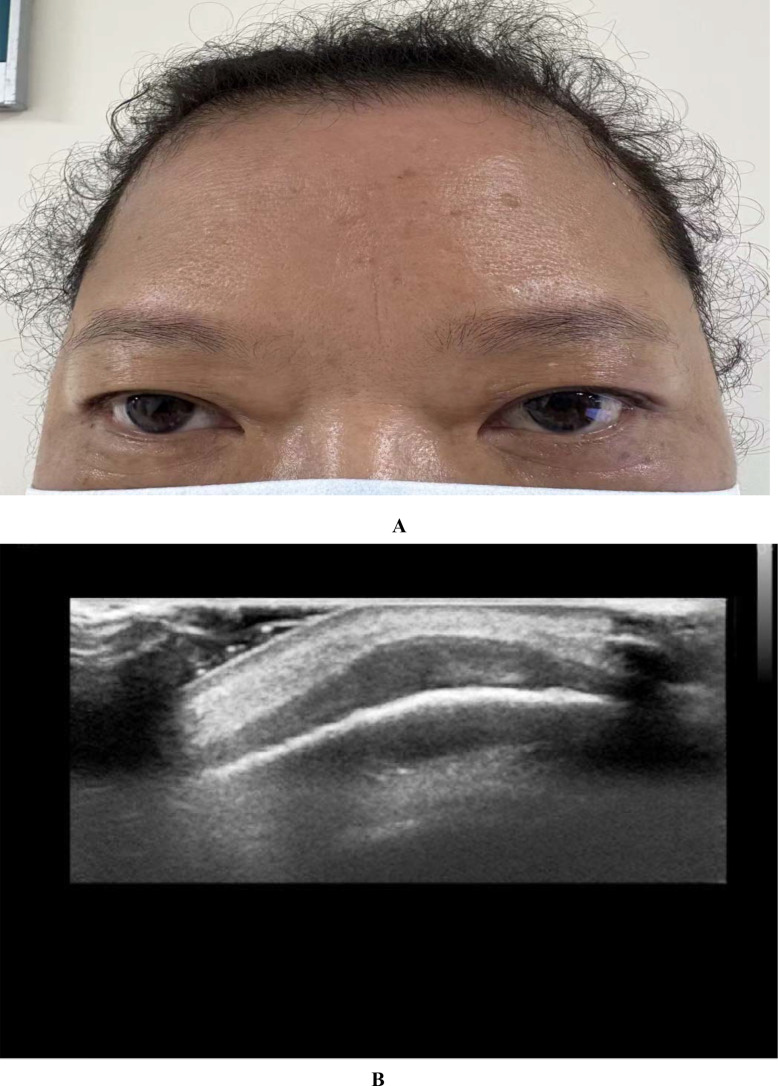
The palpable mass was almost completely resolved, and the edema around the right eyelid disappeared, with significant improvement in symptoms (A). After 4 cycles of Fulvestrant combined with Darsalizumab treatment, a follow-up superficial tissue ultrasound showed thickening of the subcutaneous layer in the left frontal area, with a maximum thickness of approximately 2.9 mm. The echogenicity was slightly reduced, and no significant blood flow signals were observed (B).
